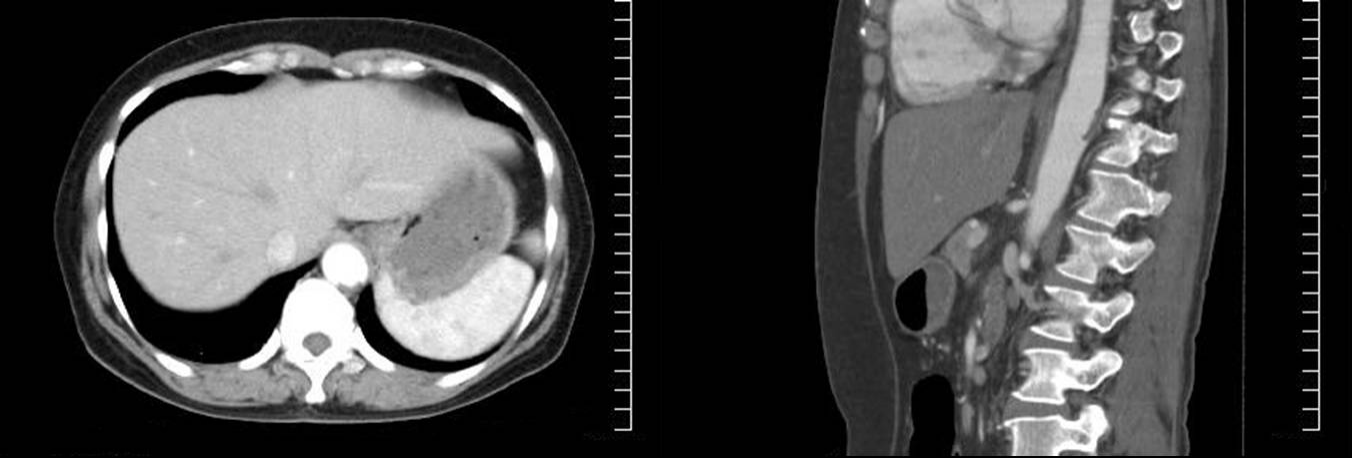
Enhanced computed tomography scan after seven days of treatment. The circular thrombus has disappeared. However, there is a low density area in the same spot, which was identified as a wall thrombosis.
